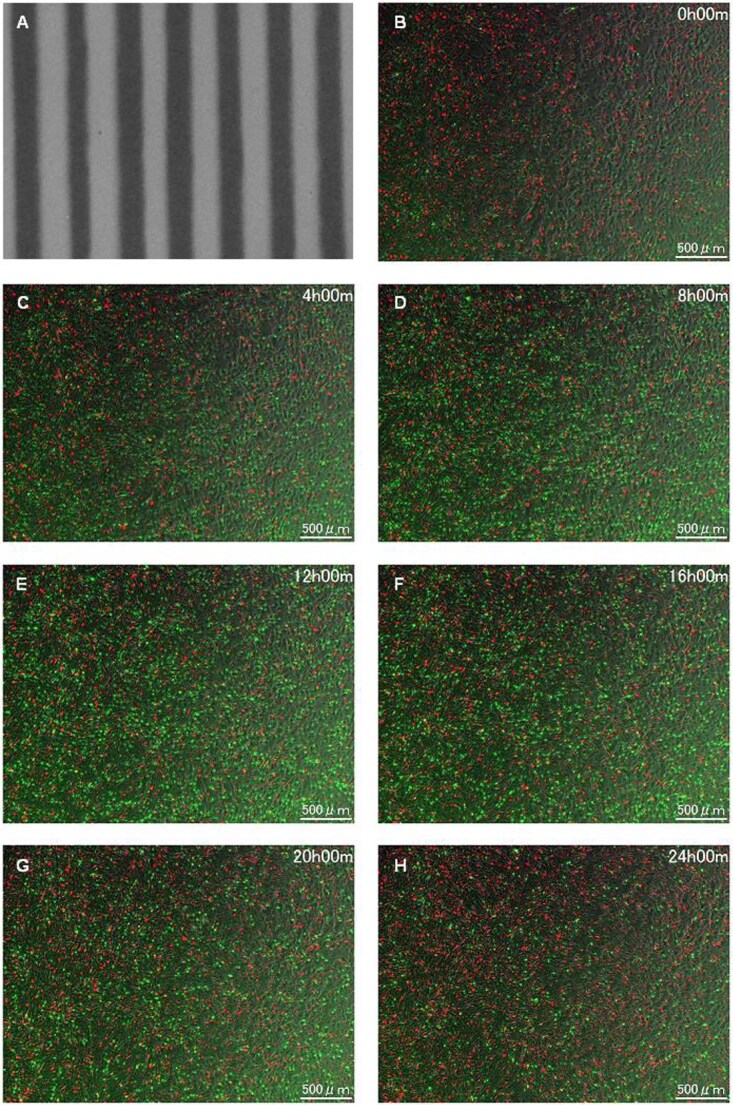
Time-lapse imaging on BJ-1-hTERT-FUCCI cells following exposure to spatially fractionated X-rays. (A) Dose profiles of the 300-μm-slit field using a Gafchromic XR-RV3 radiochromic film. (B–H) Representative fluorescent images of BJ-1-hTERT-FUCCI cells observed 4× magnification objective lens at 0 (B), 4 (C), 8 (D), 12 (E), 16 (F), 20 (G) and 24 h following exposure to the 300-μm-slit X-rays (H). Cell nuclei are indicated by the red, yellow, green and colorless regions depending on their cell-cycle phase, G1, G1/S, S/G2 and M, respectively. Scale bar—500 μm. In this study, the reproducibility of imaging results was confirmed from three or more independent experiments performed on different days.
